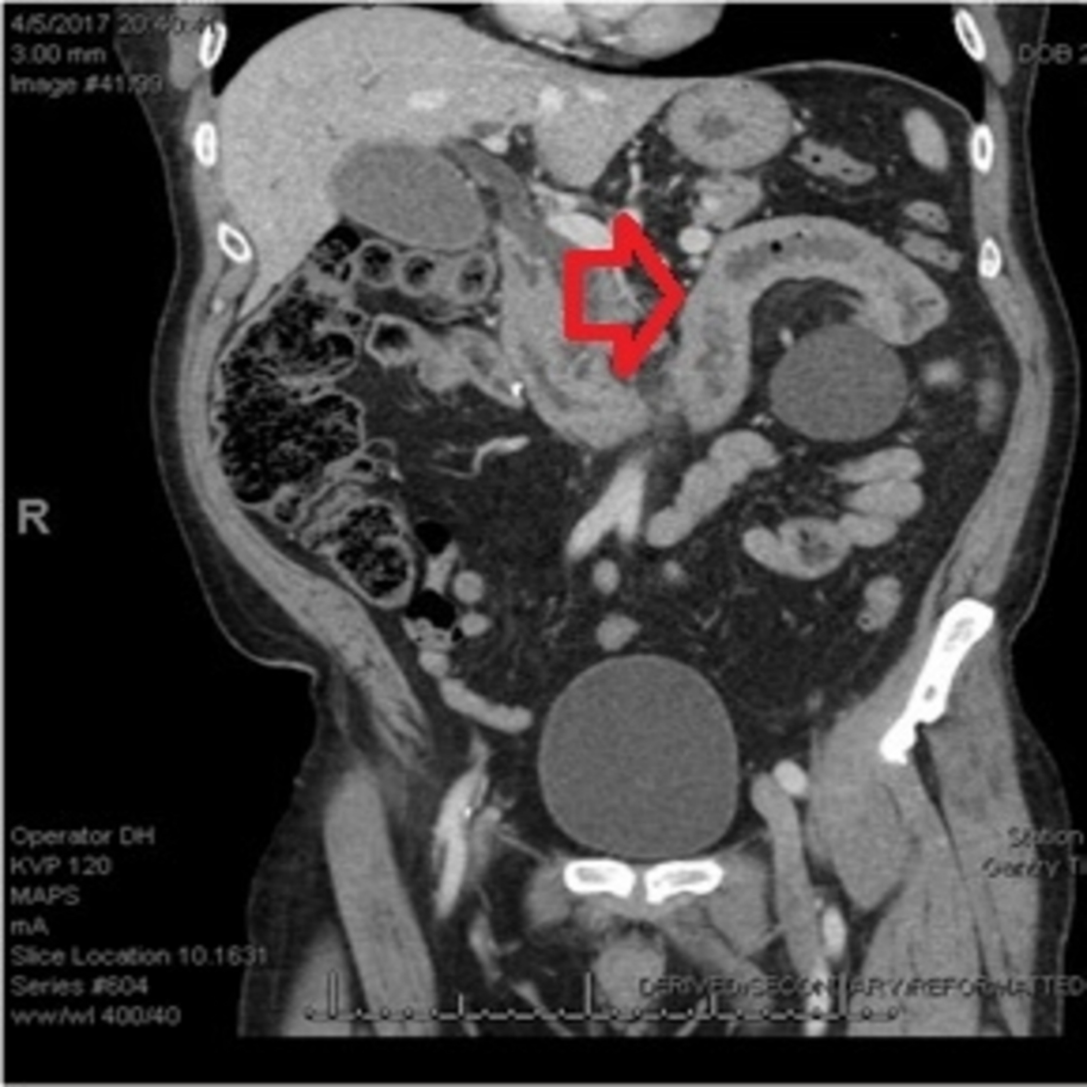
CT abdomen-pelvis showing thickening of the duodenum and proximal small bowel computed tomography (CT)
